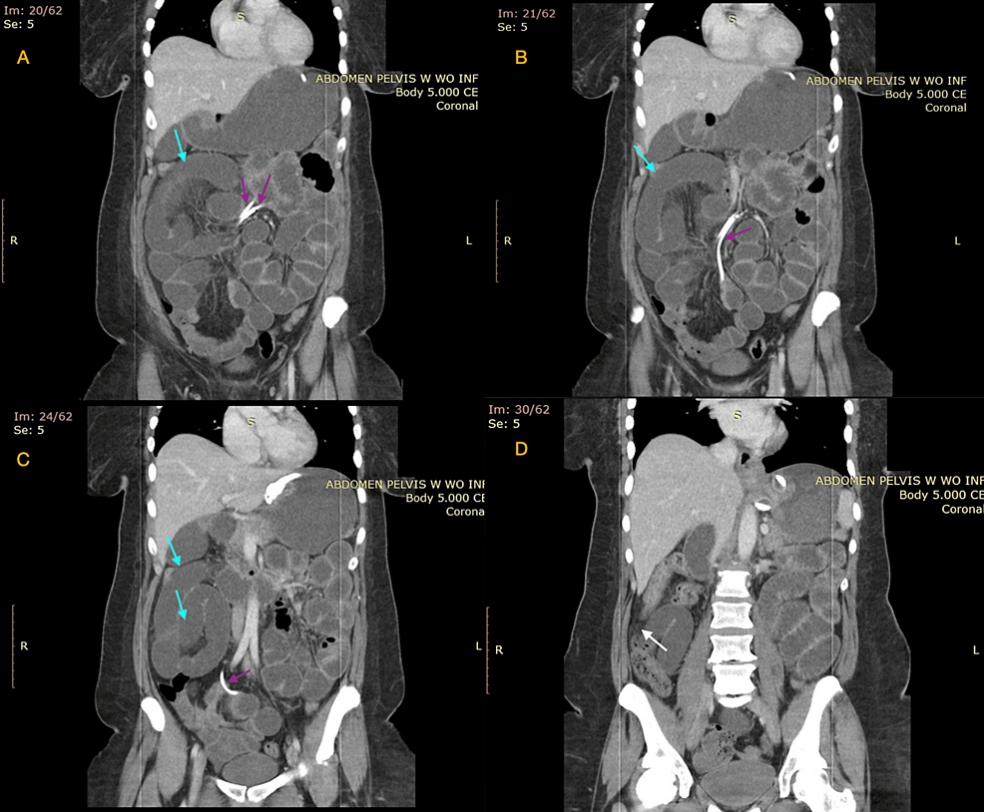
Abdominal non-contrast CT in the coronal plane (A-D) demonstrating multiple, dilated small bowel loops Figures A-C demonstrate the path taken by the LAGB connecting tube to intertwine with the mesenteric circulation (purple arrows), as well as multiple loops of dilated small bowel (blue arrows). Figure D demonstrates the transition point between the small bowel obstruction and the normal distal bowel (white arrow). LAGB: laparoscopic adjustable gastric banding
